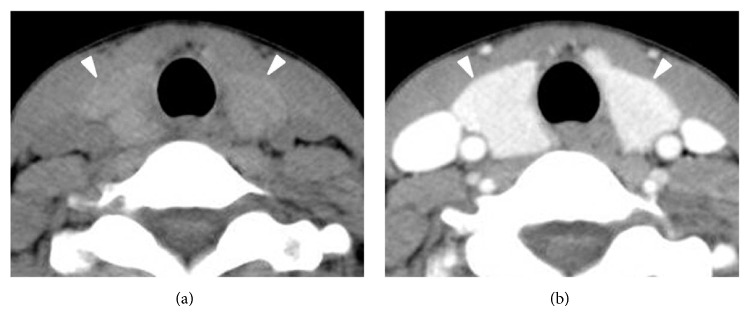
Non-Hashimoto lymphocytic thyroiditis designated “3 or more” CT classification in a 32-year-old woman (papillary thyroid carcinoma in the isthmus). The thyroid gland shows low and inhomogeneous attenuation in nonenhanced CT image (a) and mildly increased glandular size in contrast-enhanced CT image (b).
